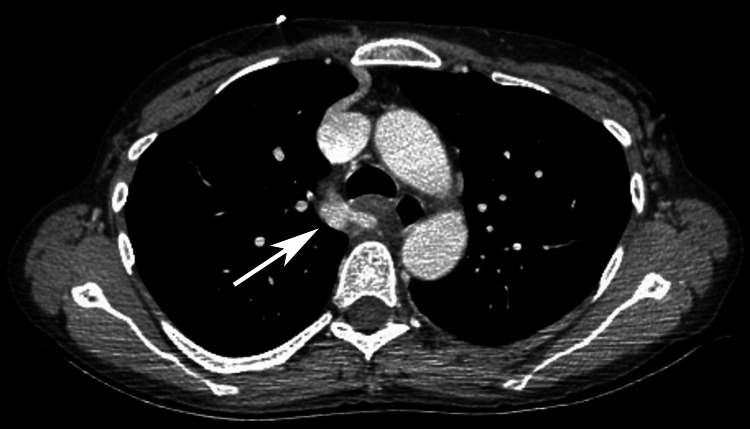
CT of the chest with IV contrast on the day of injury showing azygos vein pseudoaneurysm.
